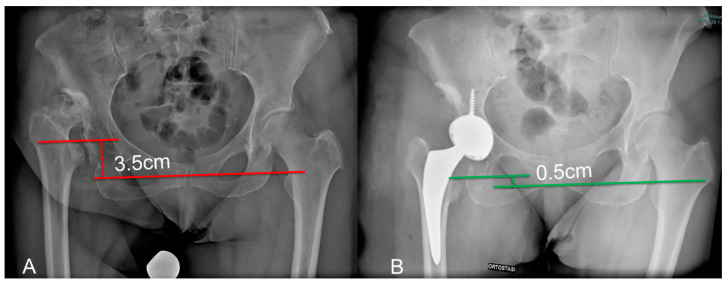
Pre-operative (A) and post-operative (B) X-rays showing a reduction in anatomical LLD referenced to the lesser trochanter.
